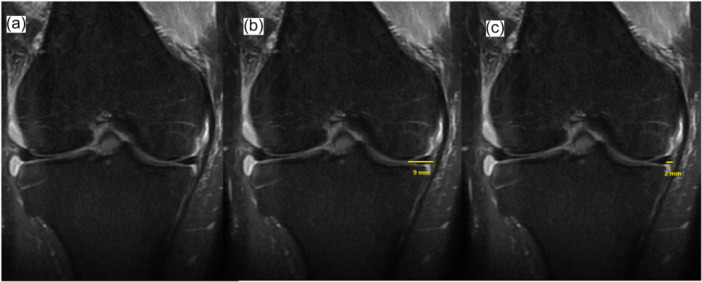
(a) Coronal MRI with a medial meniscal extrusion; (b) medial meniscal width; (c) overhanging portion of the meniscus. MRI, magnetic resonance imaging.
